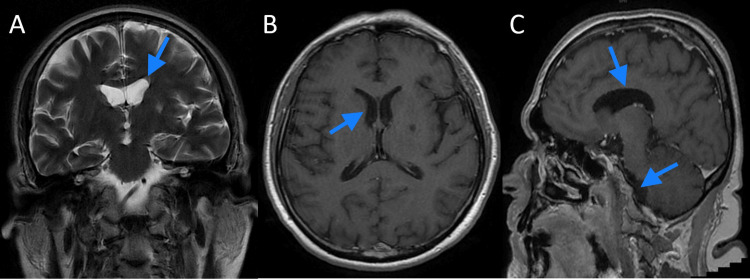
Cranial magnetic resonance imaging of the patient with contrast A: Coronal cut T2 sequence; B: axial cut T1 sequence with contrast; (C) sagittal cut T1` sequence with contrast. Blue arrows show ventricles without evidence of hydrocephalus. Courtesy of ZHUMC radiology department, Beirut, Lebanon.
